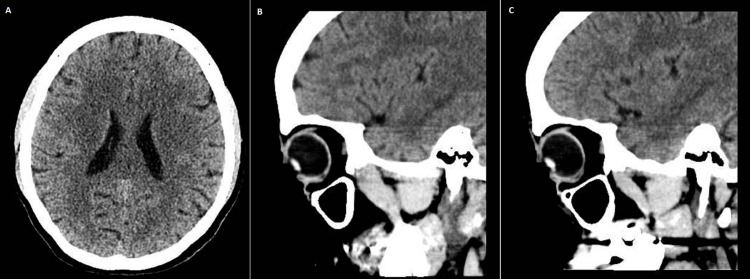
Brain (A), right orbit (B), and left orbit (C) CT scan with no abnormalities CT: computed tomography
